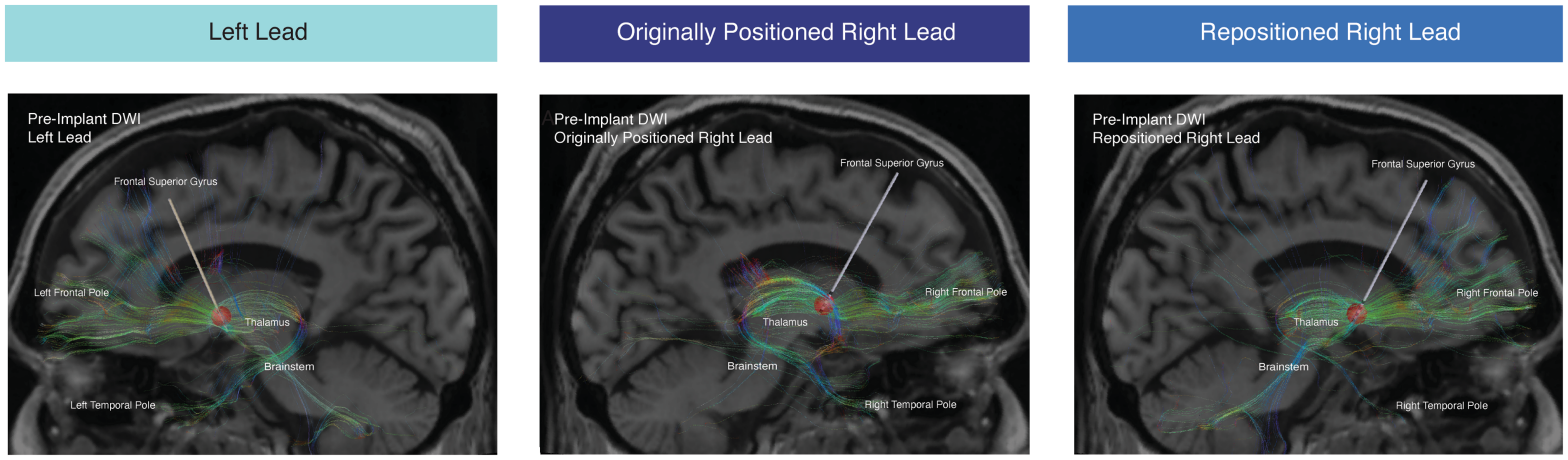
Tractography from estimated VAT. Tractography from the estimated VAT from the therapeutic active left contact (5 mA C + 2-) (left panel), right contact (5 mA C + 1-) from the originally positioned right lead (middle panel), and right contact from the repositioned right lead (right panel). Tractography was conducted with the pre-implant DWI.
